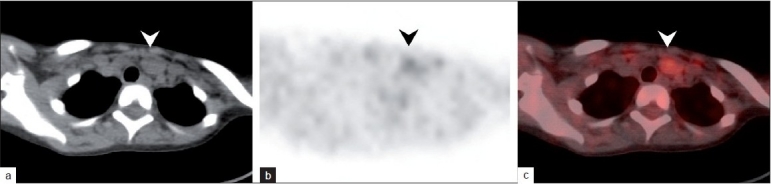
Axial CT, PET and fusion images from the follow up PET/CT
